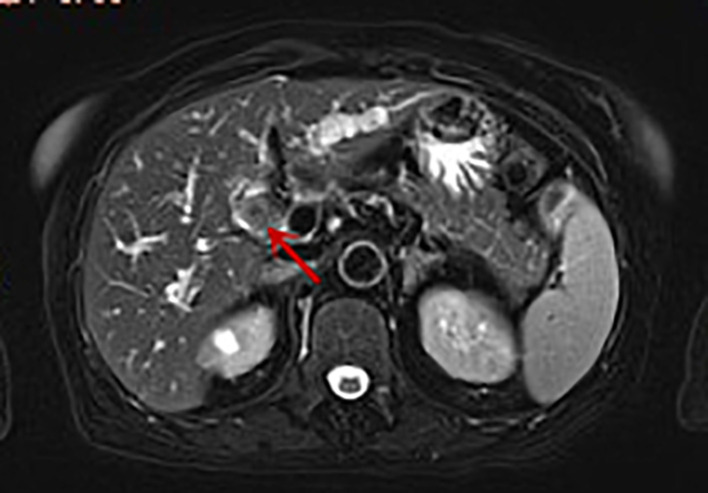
Enhanced MRI image of the patient: soft tissue shadow (indicated by the arrow) measuring approximately 1.5 × 1.8 cm can be seen in the hepatic hilum. T2 image: the tumor appears as a slightly high-signal-intensity mass.
